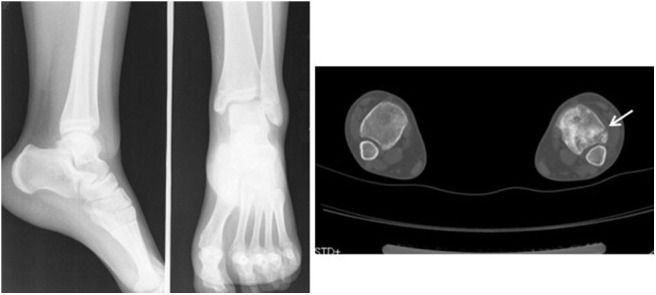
Standard radiographs of the ankle in AP and LL projections did not reveal a fracture. CT showed a small fracture in the distal fibula (arrow).
